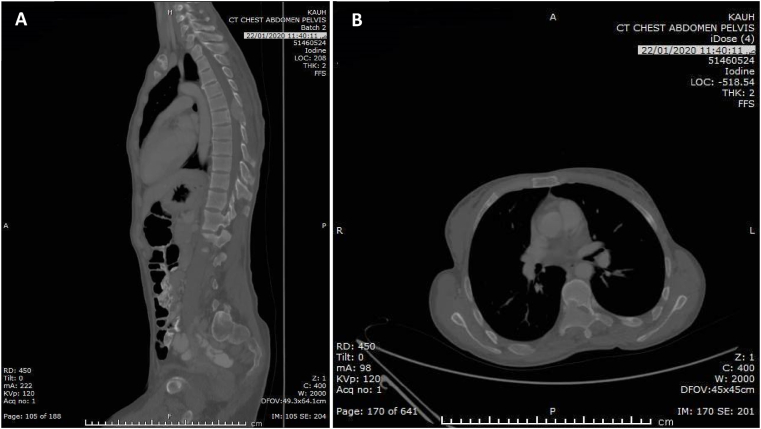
(A) sagittal chest abdomen pelvis CT showing expansile bony mass involving the body, right pedicles and posterior arch of T7. (B) axial T7 CT showing epidural soft tissue component extending from T6-T7 compressing the spinal canal, dislocating its content to the left and scalloping the T7 vertebral body.
